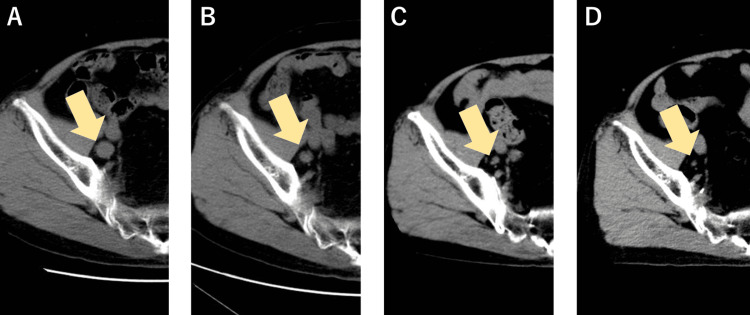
Time course of axial CT scan of the pelvis: (A) November 2012 (PSA:8.36 ng/ml), (B) December 2013 (PSA: 3.5), (C) February 2015 (PSA: 1.8), and (D) August 2018 (PSA: 0.01). PSA: prostate-specific antigen
